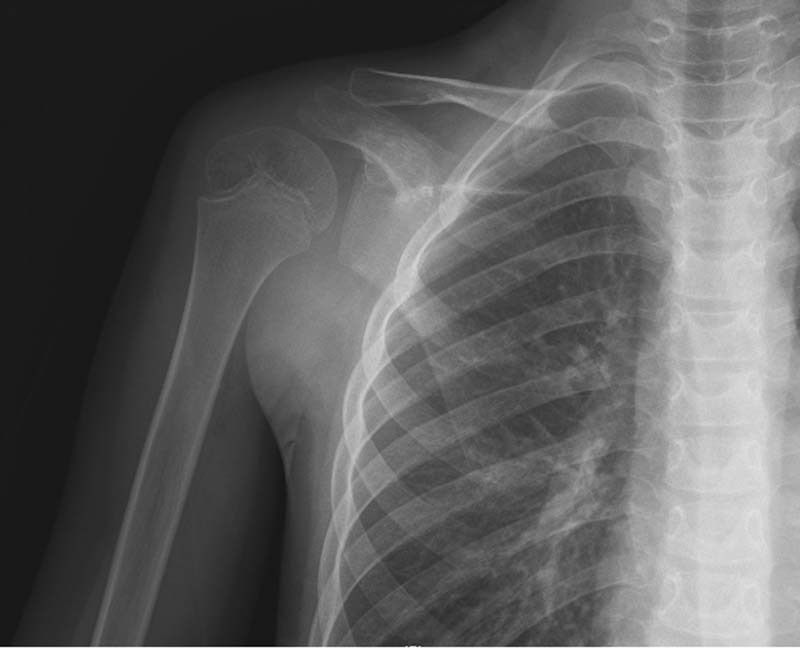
Anteroposterior (AP) X-ray of the right shoulder at initial presentation demonstrating no obvious deformity, dislocation, or malignancy.
